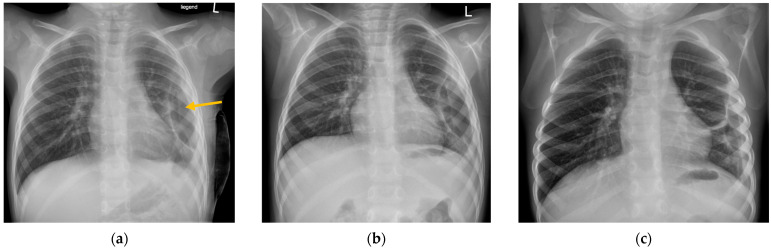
“Pneumothorax ex vacuo” in a 2-year-old girl. (a) Anterior–posterior (AP) chest radiograph taken 2 days after discharge showing a left-sided pneumothorax (arrow). (b) Persistent pneumothorax 7 days after discharge. (c) At 2.5 weeks, pneumothorax is still radiologically detectable.
